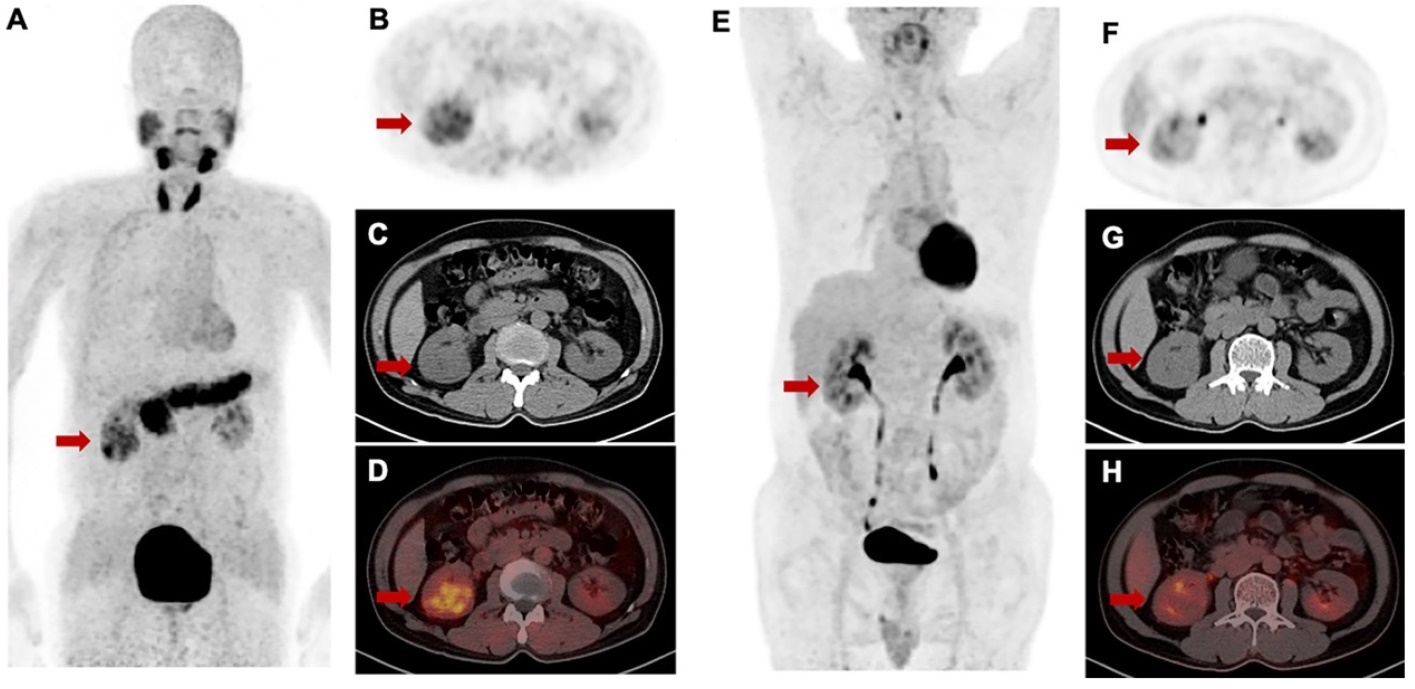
Intra-individual comparison of [^68^Ga]Ga-FAPI-RGD (A-D) and 2-[^18^F]FDG (E-H) in a patient with renal cell carcinoma. [^68^Ga]Ga-FAPI-RGD showed intense uptake in the primary lesion (SUVmax = 5.1), while there was only slight uptake on 2-[^18^F]FDG PET/CT (SUVmax = 2.7).
